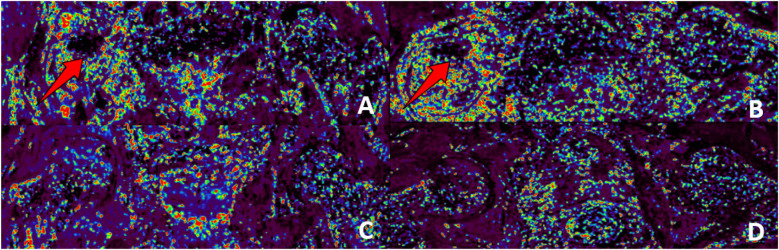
Preoperative and 6-month postoperative DCE-MRI images of the right femoral head. (A,B) Preoperative coronal and axial scans reveal substantial aberrant hyperperfusion in the right femoral head; (C,D) Postoperative coronal and axial scans showed a significant reduction in the hyperperfusion phenomena of the right femoral head, but blood perfusion in the collapsed area (shown by the arrow) has increased, suggesting partial restoration of vascular function in that region. Furthermore, the postoperative scans indicate a distinct boundary of the femoral head and a reduction in the edema of the surrounding tissues. The aforementioned results indicate that the aberrant hyperperfusion of the right femoral head is partially rectified, and the blood supply is restored post-surgery.
